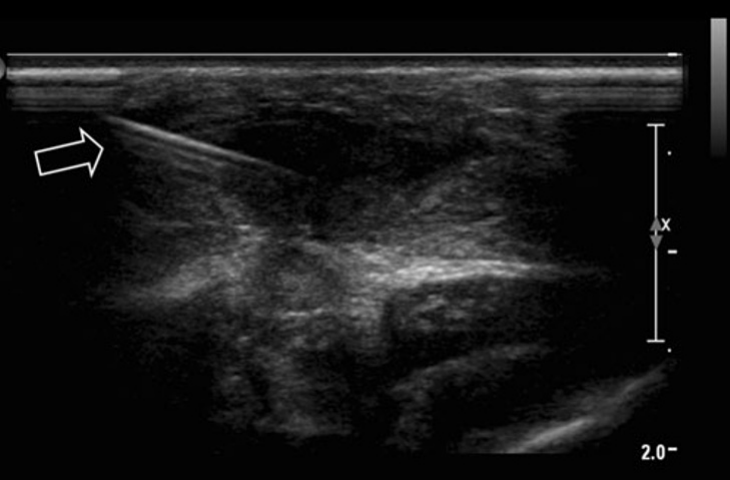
Ultrasound-guided biopsie needle aspiration of a subcutaneous pre-sternal abscess
